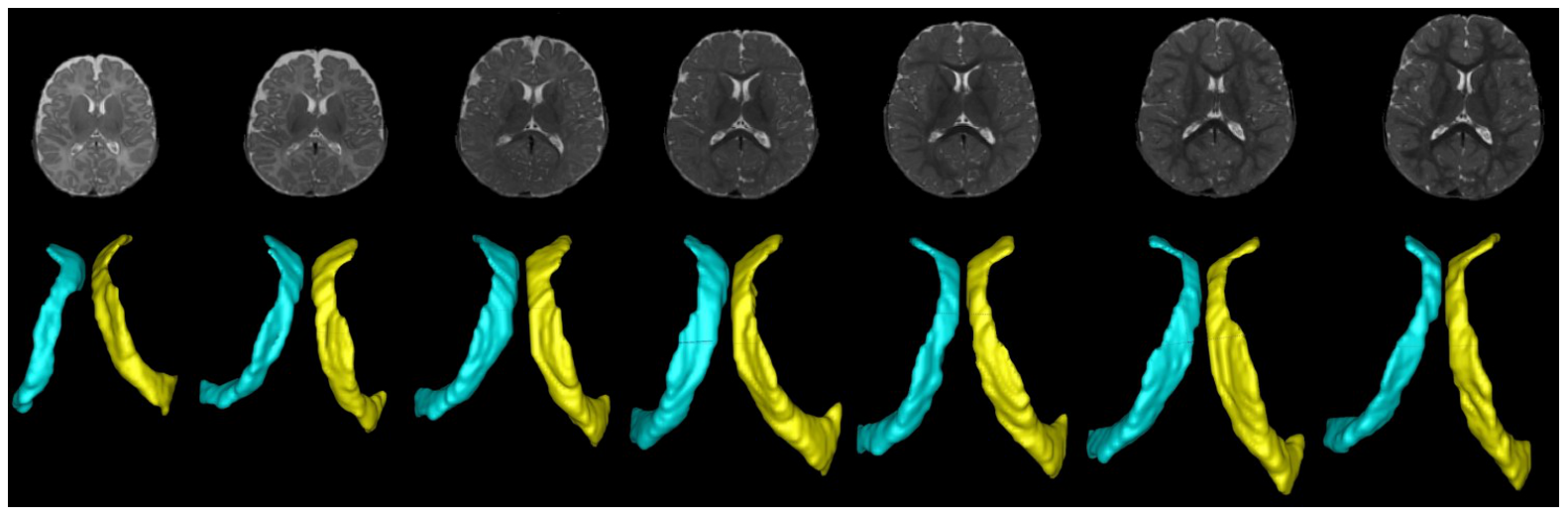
Representative T2-weighted images (upper row) from a subject imaged over the course of the first two years of life along with the segmented left and right ventricles (lower row) are shown.
